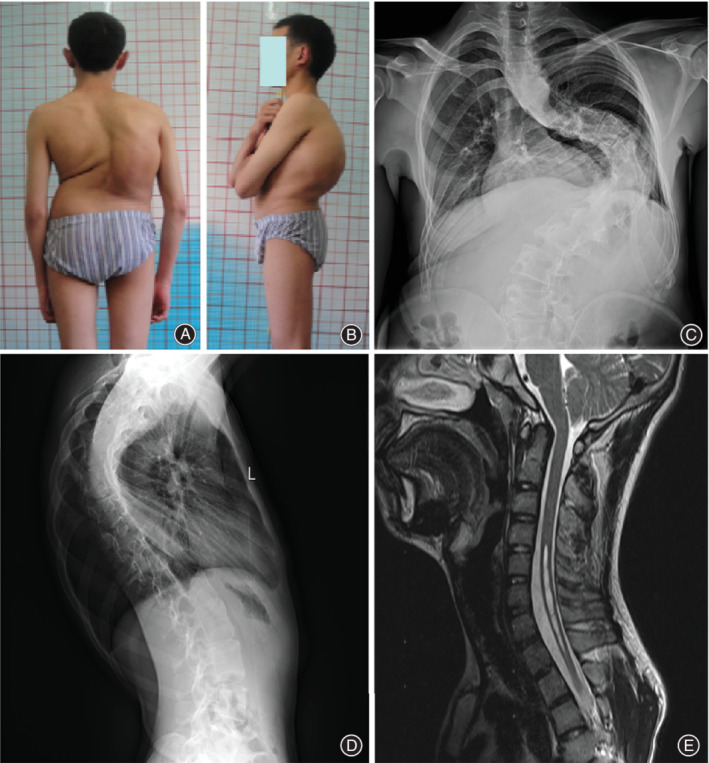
An 18‐year‐old boy with severe kyphoscoliosis associated with cervical intraspinal neural axis abnormalities (CIINAA). The preoperative appearance reveals spinal deformity (A, B). The preoperative AP and lateral radiographs show the severe kyphoscoliosis with trunk imbalance (C, D). The MRI shows the Chiari malformations and syrinx in the cervical region (E).
